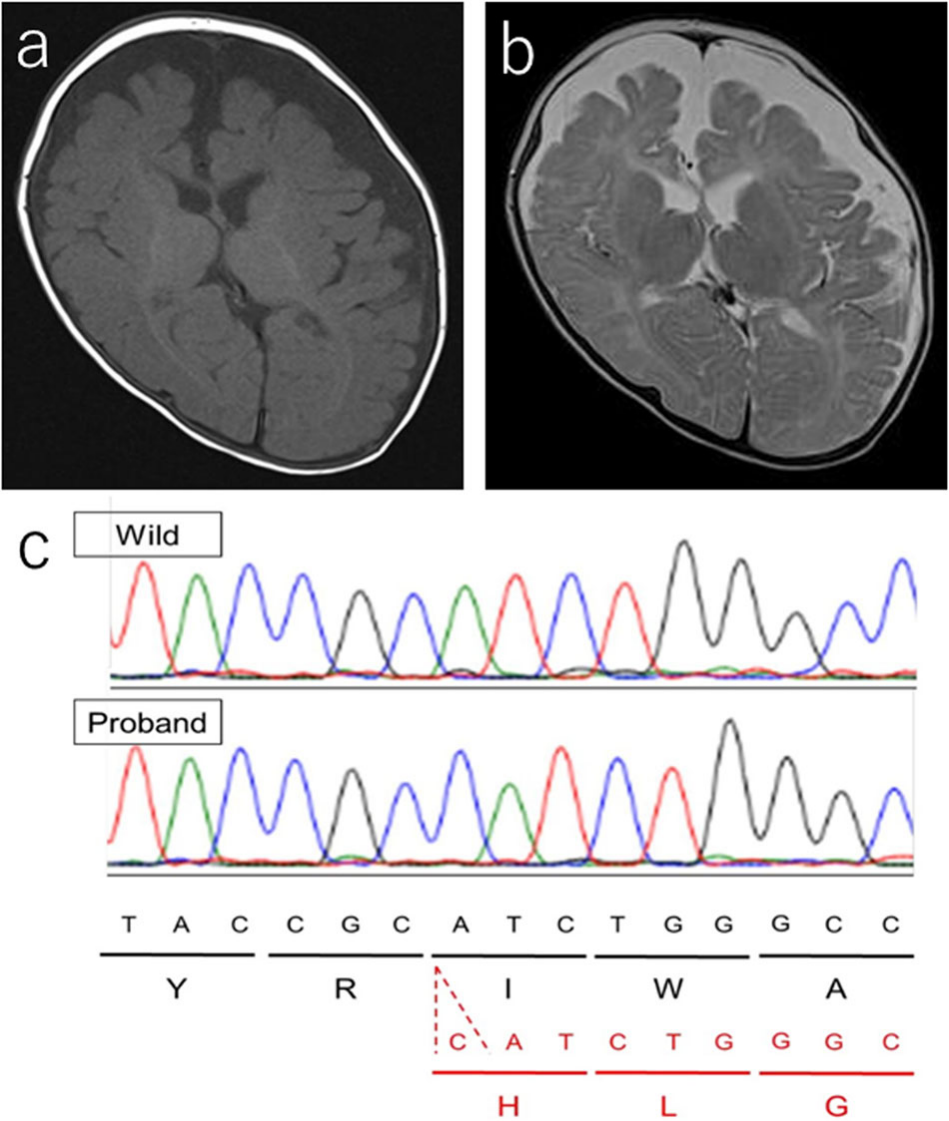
The clinical and genetic presentation of this case. Brain magnetic resonance imaging of this patient at 6 months of age (a T1-weighted axial image, b T2-weighted axial image). The anterior and posterior limbs of the internal capsule and corona radiata show a high signal intensity. On the other hand, the low signal intensity in the frontal white matter suggests delayed myelination. The expansion of the subarachnoid space indicates cerebral hemisphere atrophy. c An electropherogram showing the mutation in this case. One nucleotide duplication, c.966dup [p.(Ile323Hisfs*57)], was found in *SLC16A2* exon 3.
